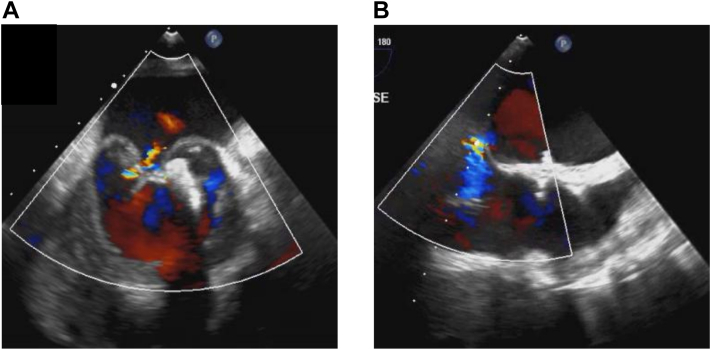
Echocardiographic Findings After Transcatheter Edge-to-Edge Repair (A) TEE image showing mild residual mitral regurgitation after transcatheter edge-to-edge repair. (B) TEE image confirming the absence of residual iatrogenic atrial septal defect following removal of the guide catheter. TEE = transesophageal echocardiography.
